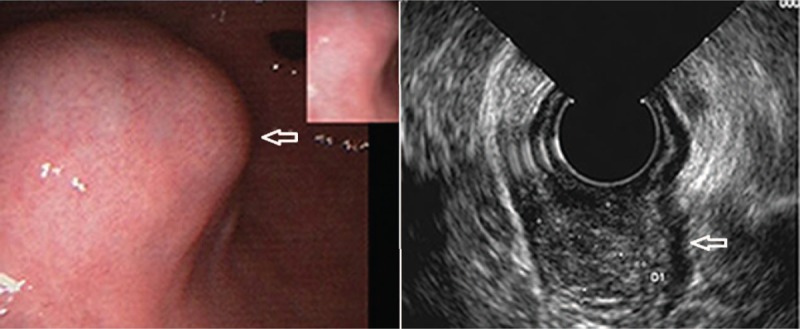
Endoscopic ultrasonography in the diagnosis of gastric stromal tumors.
